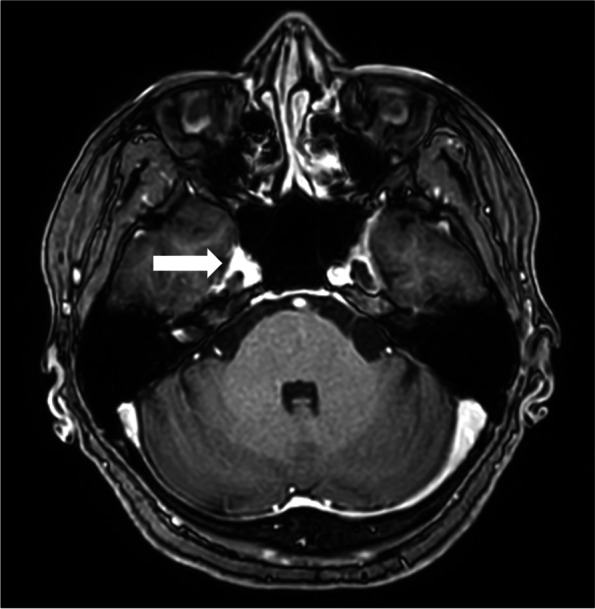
Brain magnetic resonance image. Increased enhancement in the distal right internal auditory canal is evident (arrow)
